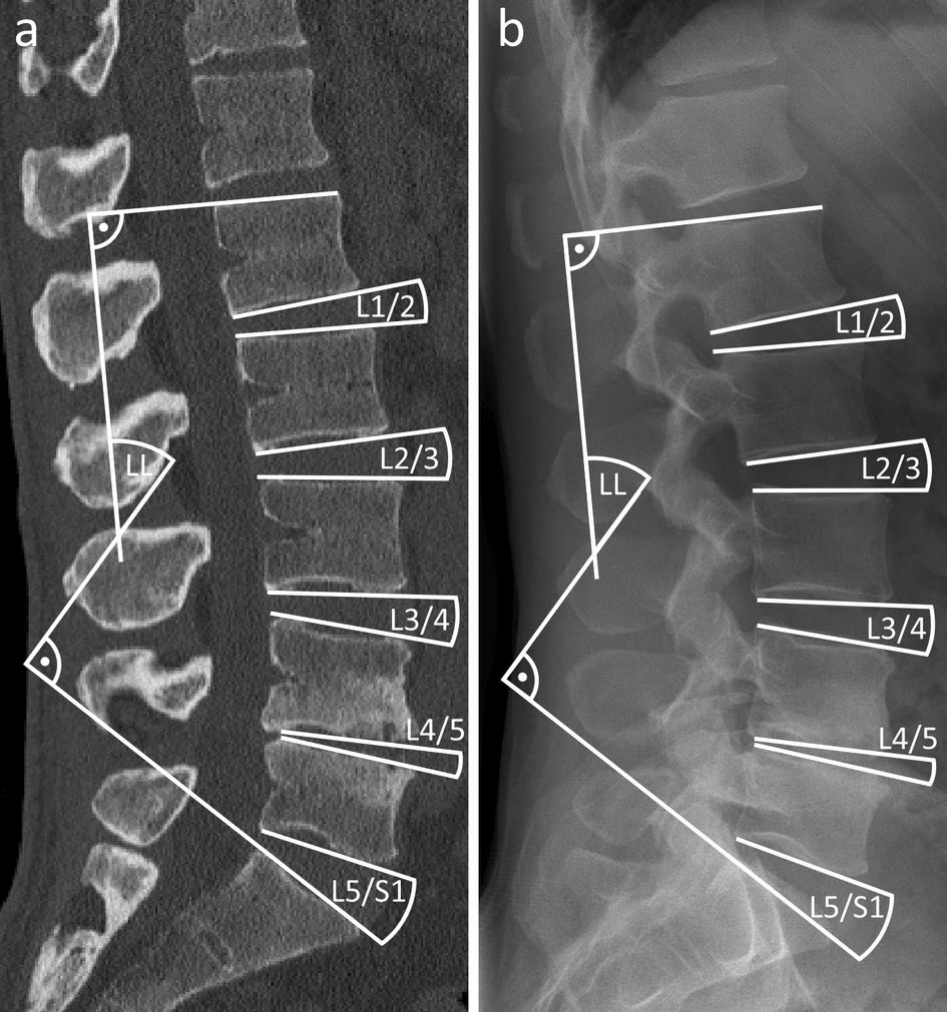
Measurement of intersegmental and LL angles in a (a) CT-scan and (b) radiograph.
